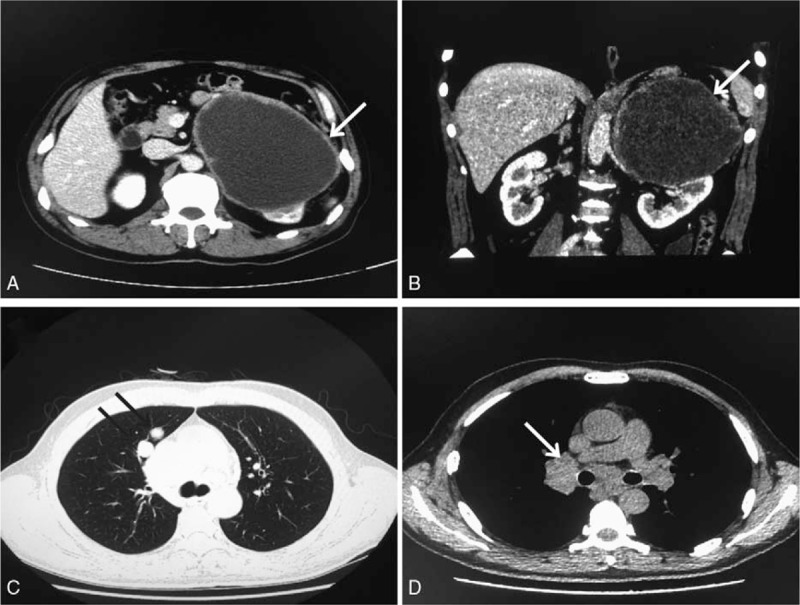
(A, B) Renal plain CT and enhancement CT scan showed a tumor in the upper region of the perinephric space. (C, D) Pulmonary CT scanned right upper lobe metastases and enlarged lymph nodes of mediastinum. CT = computed tomography.
